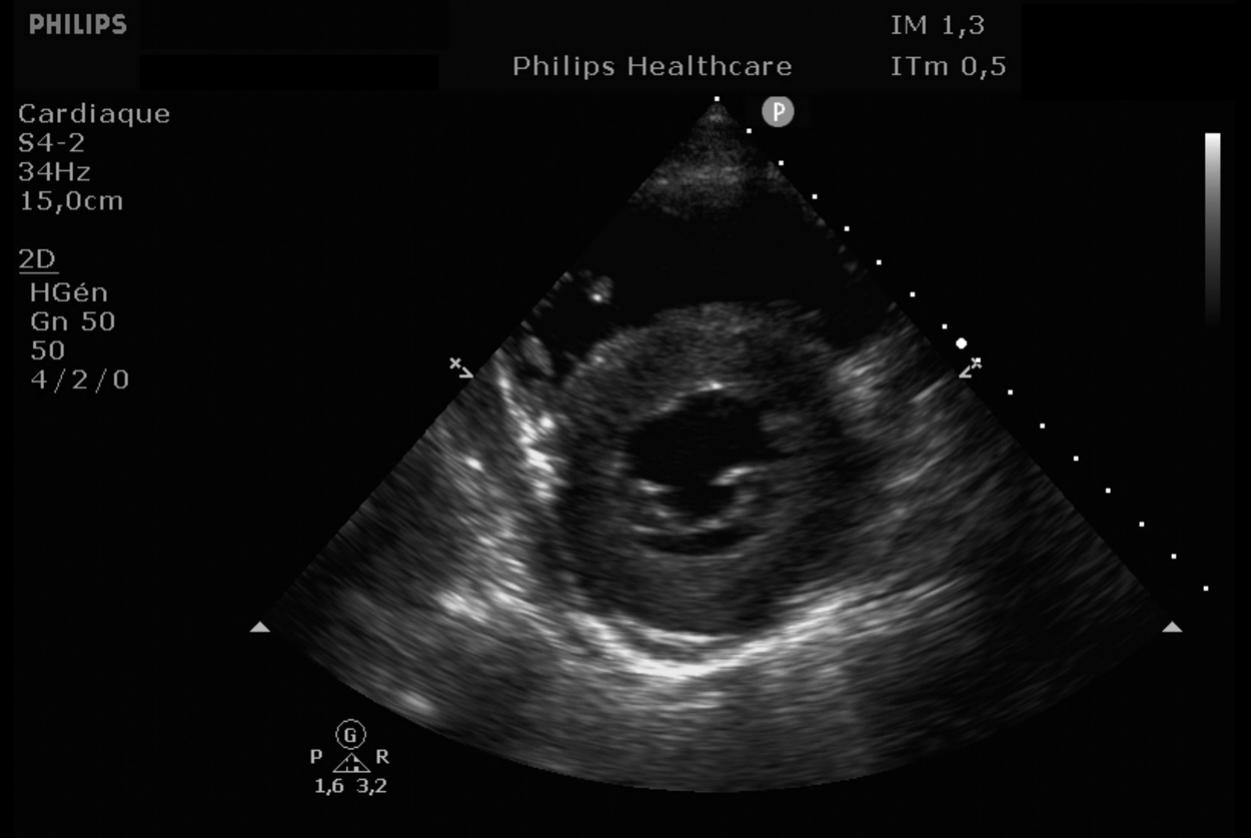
Parasternal short axis window
